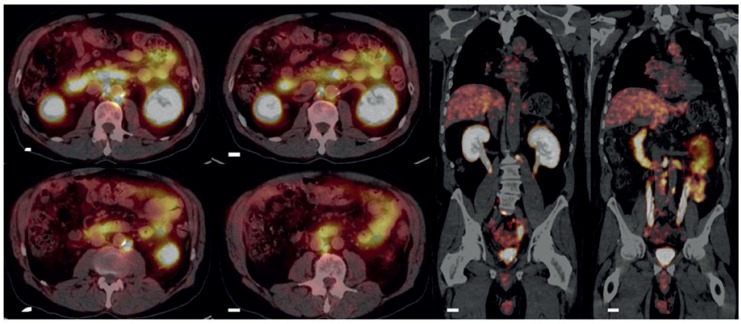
Example of patient submitted to ^68^Ga-PSMA PET/CT due to biochemical recurrence. The prior CT images and MRI performed were negative for metastases. The ^68^Ga-PSMA PET/CT images demonstrate normal size lymph nodes that are avid for ^68^Ga-pSMA.
